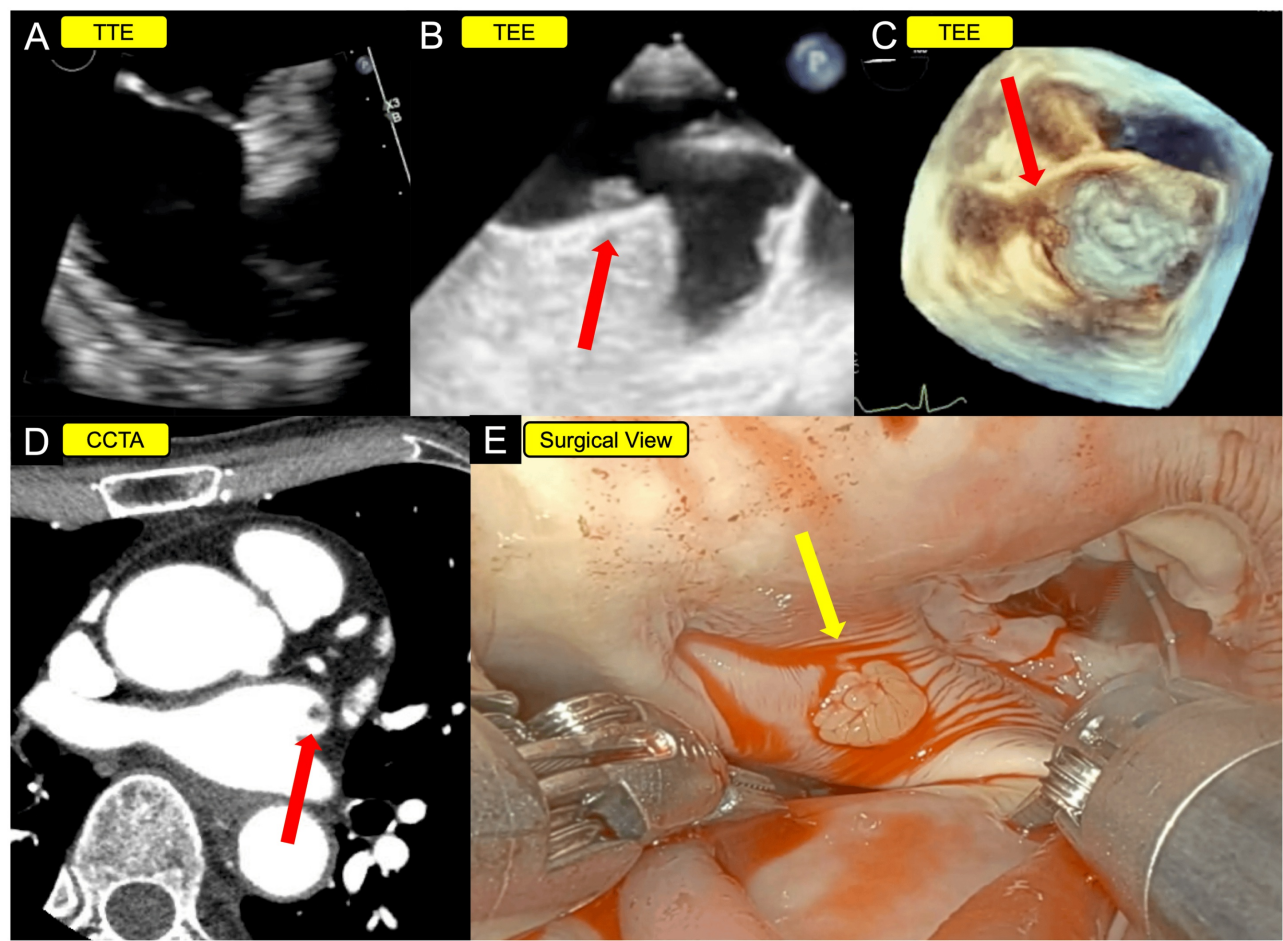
Multimodality imaging of the case (A) TTE did not reveal any obvious mass. (B, C) TEE revealed a highly mobile 5-mm mass attached to the coumadin ridge (red arrow). (D) CCTA identified a 5-mm mass near the base of the left atrial appendage (red arrow). (E) Intraoperatively, a papillary fibroelastoma was identified on the coumadin ridge (yellow arrow). TTE: transthoracic echocardiography; TEE: transesophageal echocardiography; CCTA: cardiac computed tomography angiography
